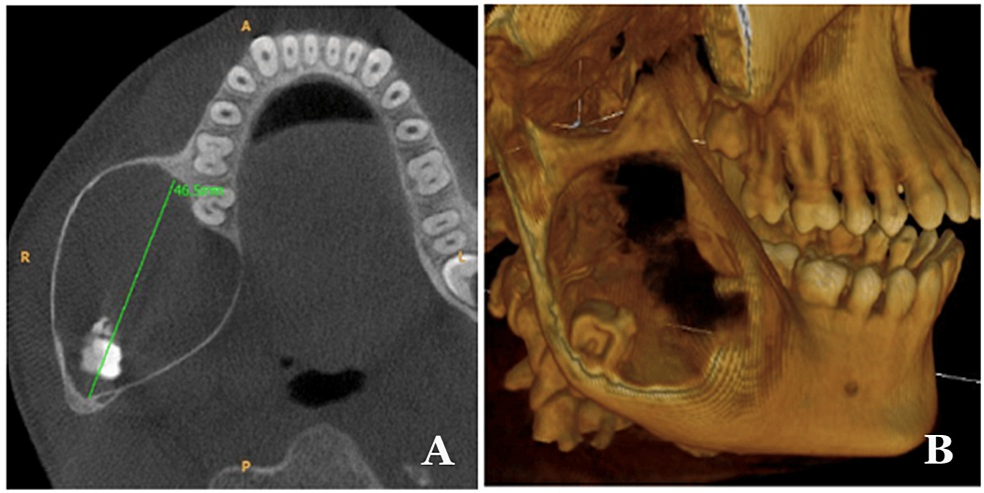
Computed tomography of the mandible showed 38.3 mm of mediolateral expansion of the cortical plates. [B] 46.5mm anteroposterior expansion of the lesion showing intact borders of the mandible
